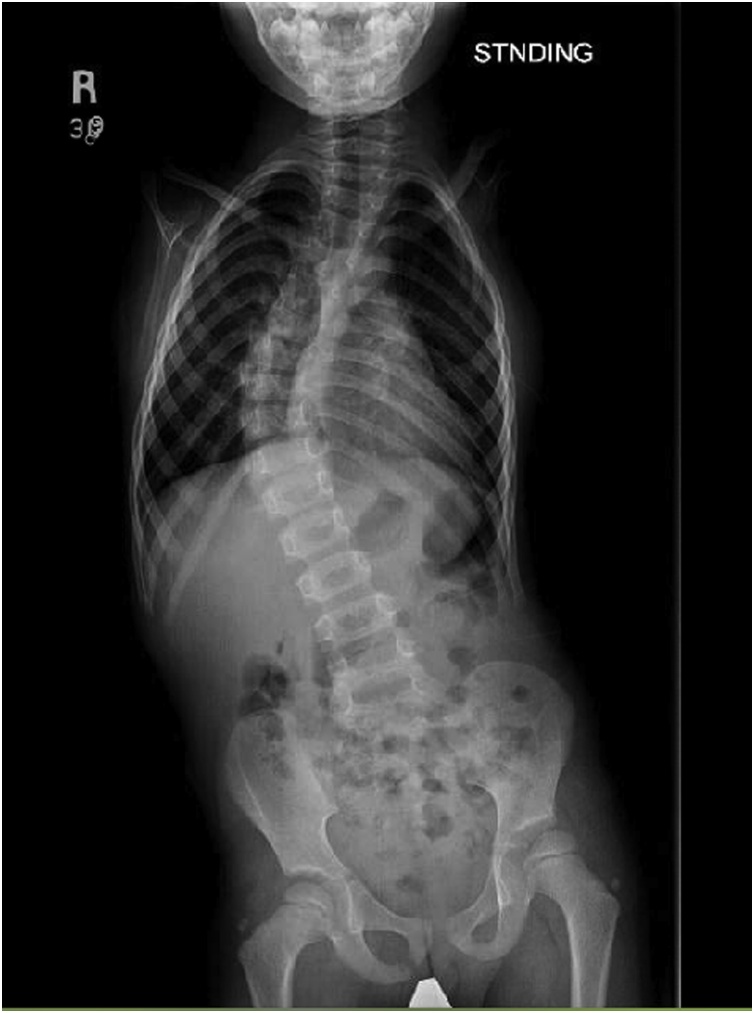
Right thoracolumbar curve of 45 degrees was identified in imaging without any other vertebral or cord abnormalities in MRI.
